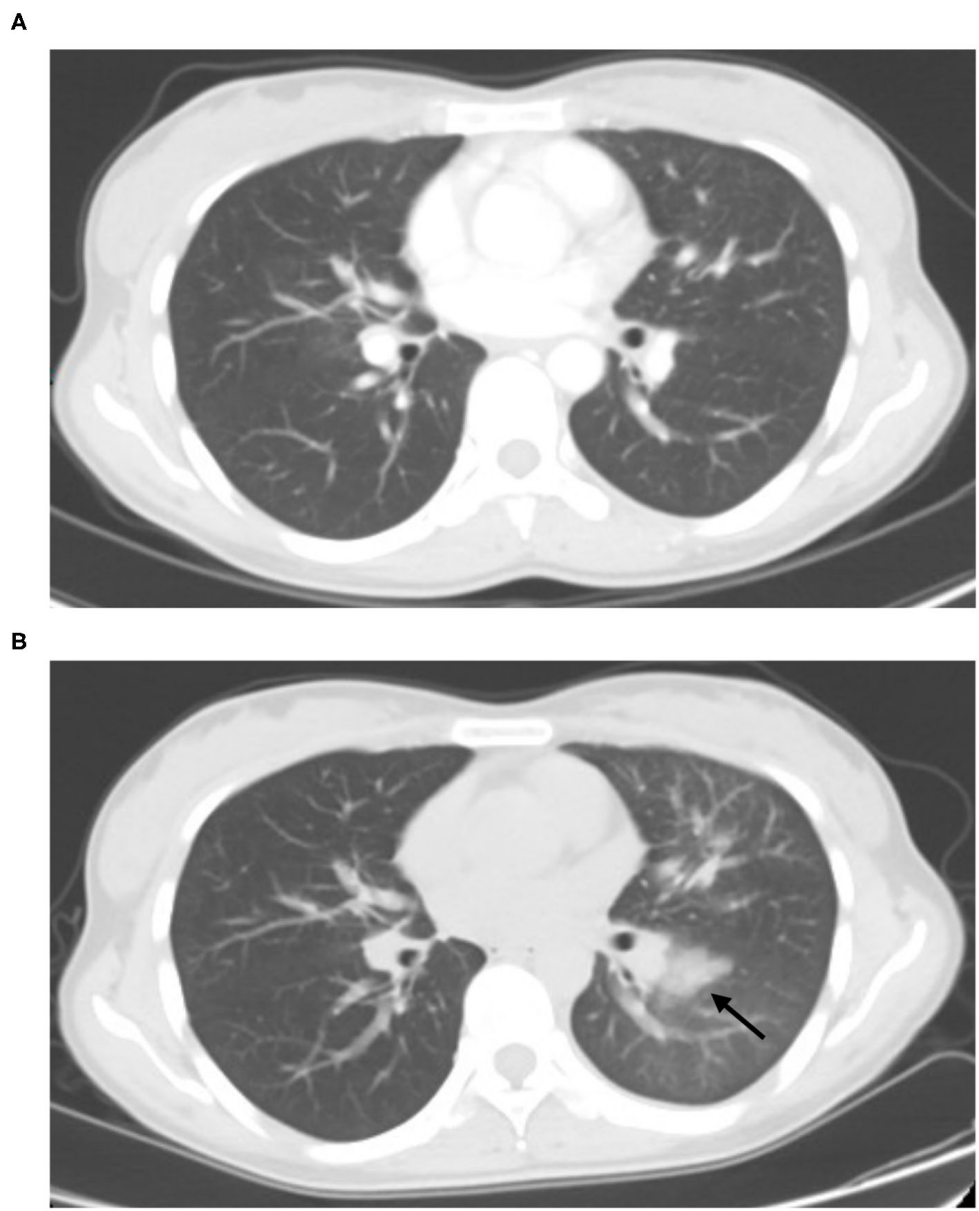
Twenty-seven-year-old female with post-chemotherapy of lung cancer history. Comparison to the prior CT scan (A) which performed at 2 months ago, the focal left lower lobe subpleural consolidations was identified [(B), arrow].
